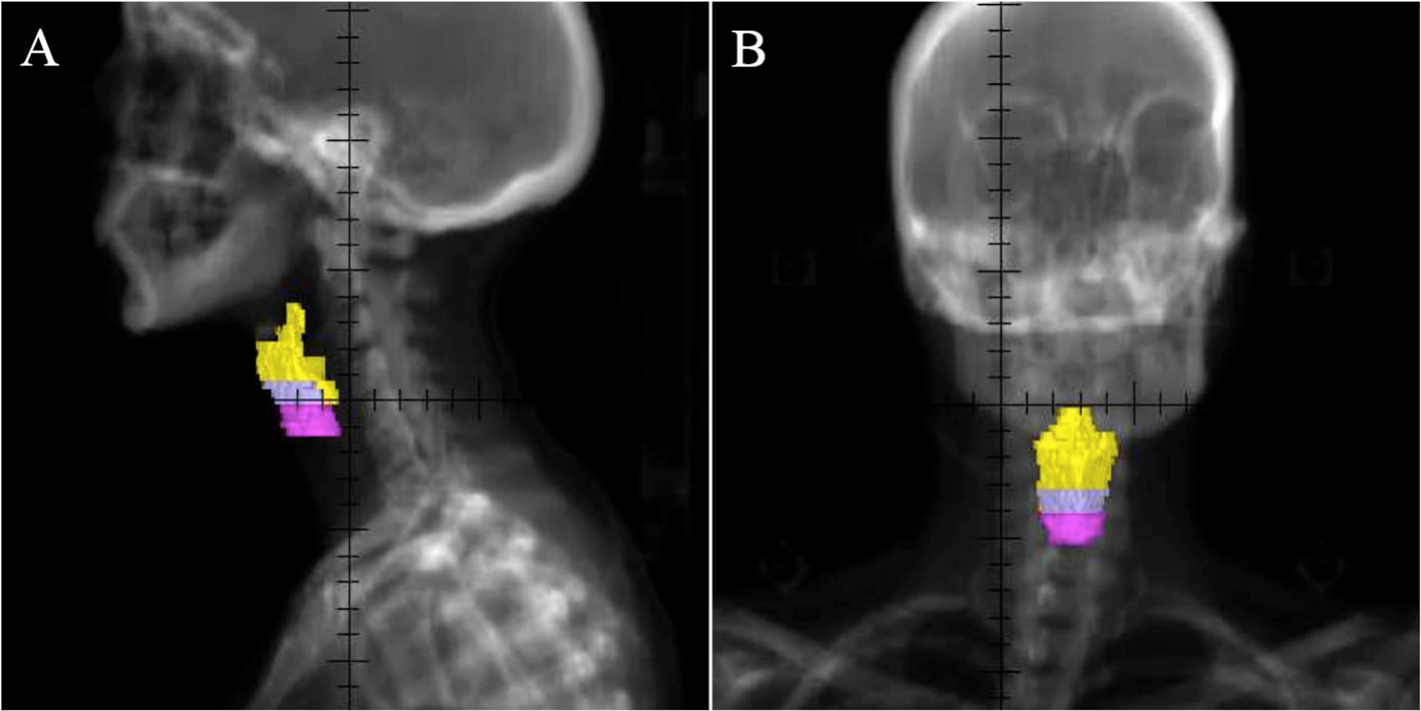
Digitally reconstructed radiographs of the major divisions of the larynx generated from contours shown in Figure
